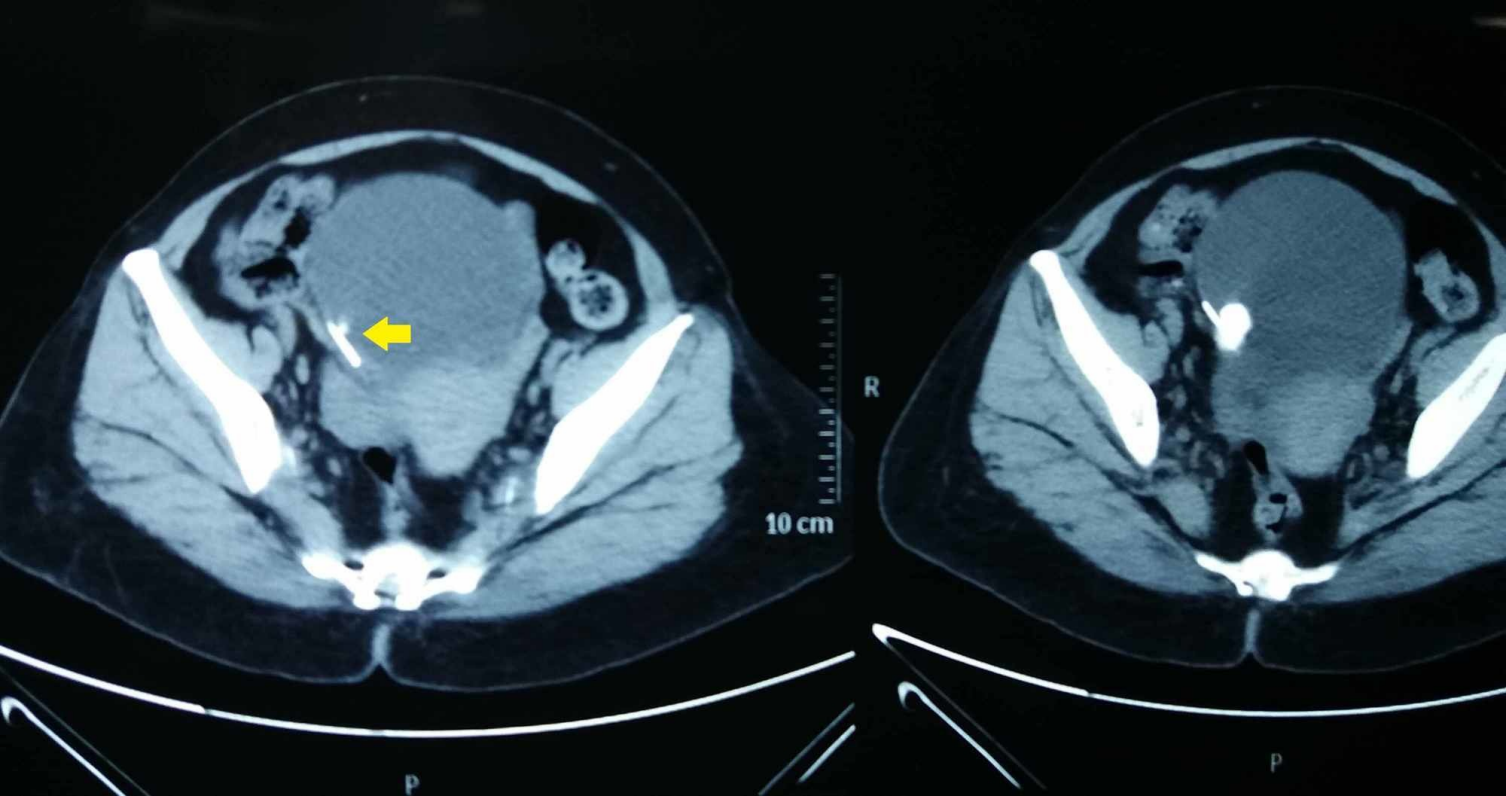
Transverse section of noncontrast computed tomography showing one of the limbs of the copper intrauterine contraceptive device (yellow arrow) embedded within the right lateral bladder wall with overlying calculus
